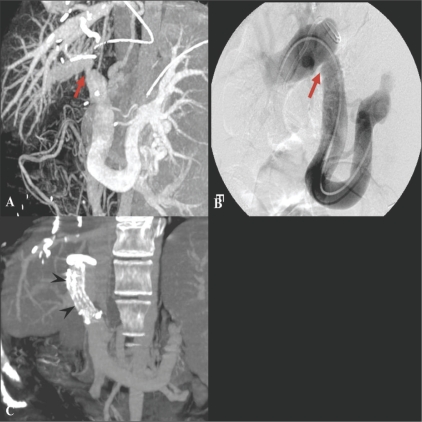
A 45-year-old woman with portal venous stenosis that occurred 8 days after liver transplantation. Coronal oblique MIP image (A) reveals a tight stenosis (arrow) at the portal vein anastomosis. Anterioposterior view of a direct main portal venogram (B) confirms the stenosis (arrow). The stenosis disappeared following metallic stent placement. Follow-up coronal MIP CT scan image (C) shows a patent stent (arrowheads) in the main portal vein
